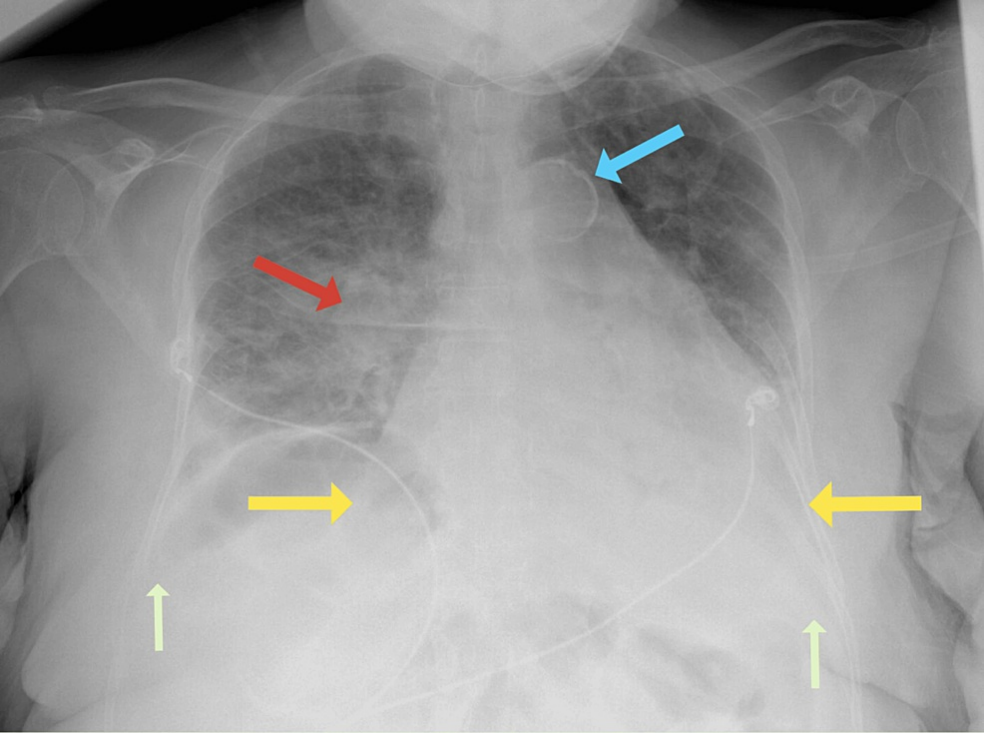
Chest radiography at the time of admission. Enlarged cardiac silhouette (yellow arrows). Calcified aorta (blue arrow). Increased central perihilar pulmonary vascular congestion (red arrow). Pleural effusions (left greater than right) are not excluded (green arrows). Decreased inspiratory effort. No pneumothorax.
